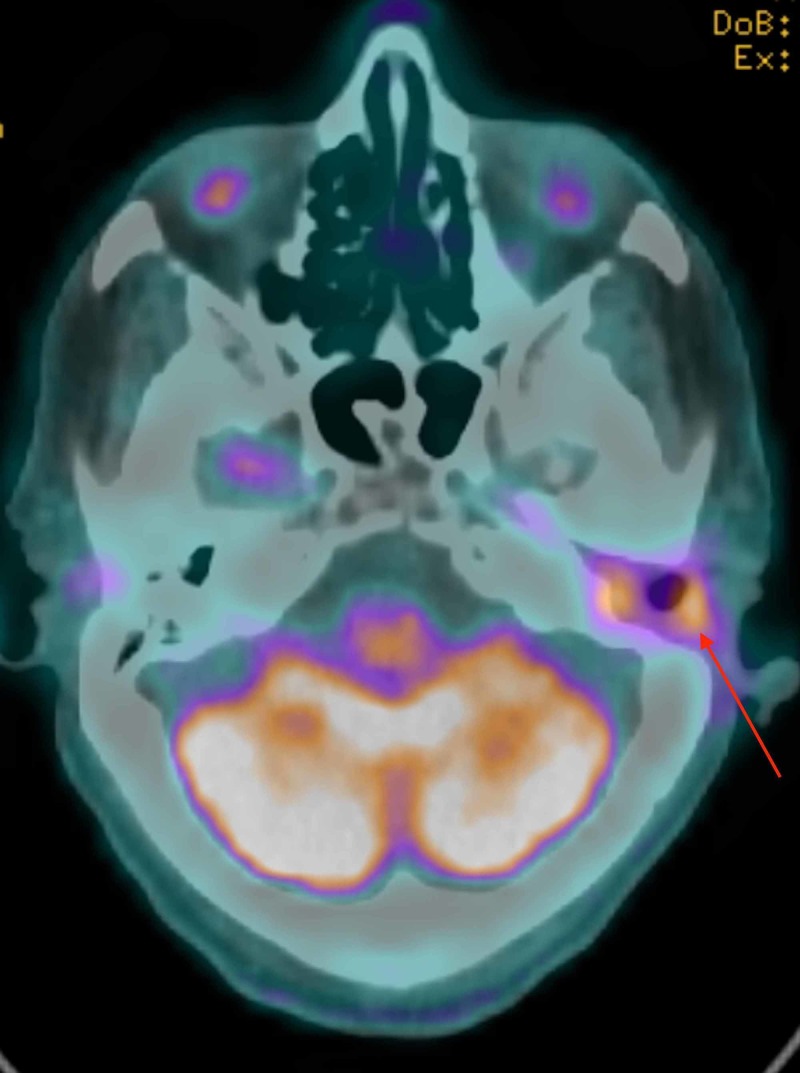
Axial head PET-CT shows FDG avid lesion located in the left external ear canal suggestive of residual active disease. FDG: Fluorodeoxyglucose
